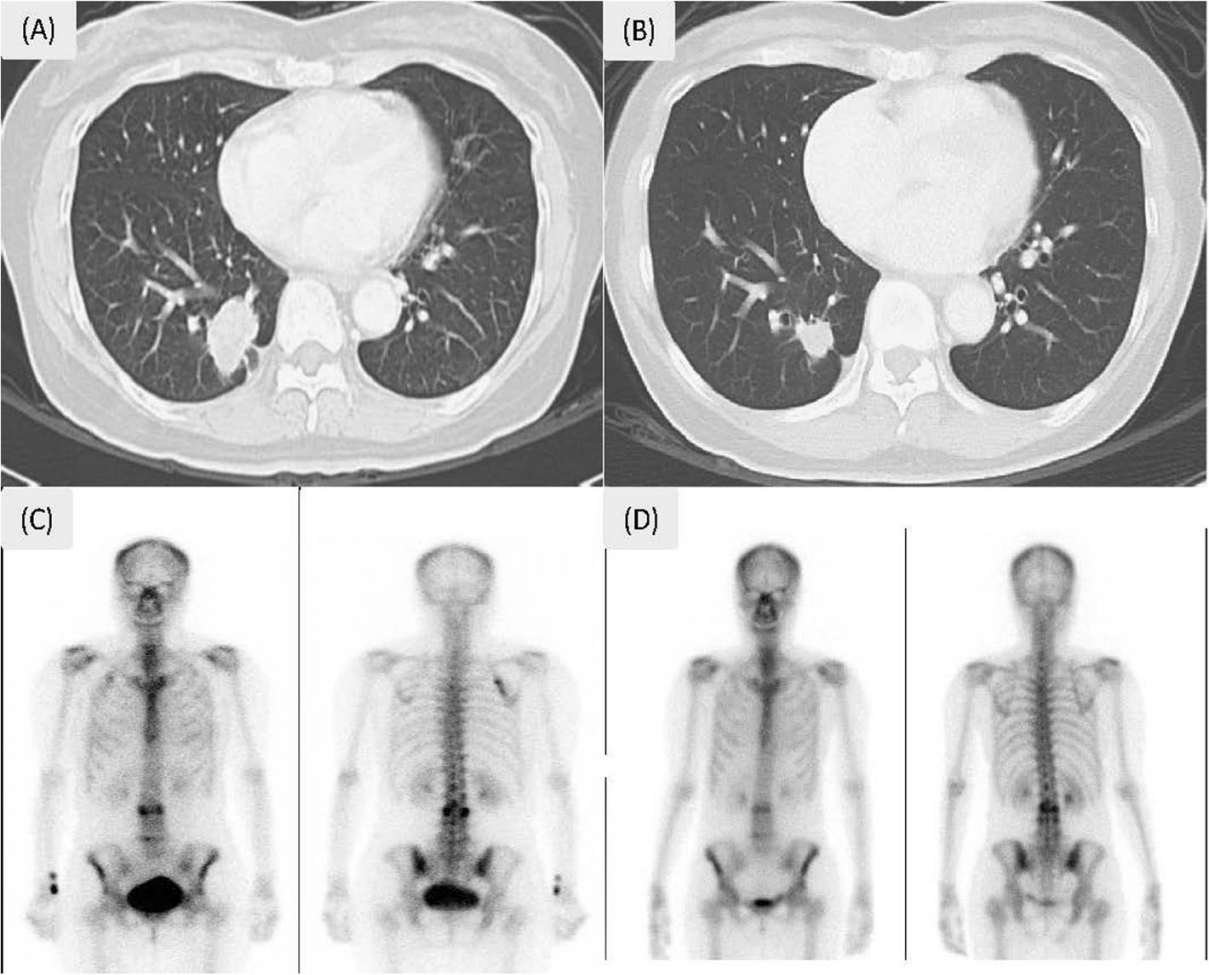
Patient scans. a Chest computed tomography scan: pre-tyrosine kinase inhibitors (TKI) therapy in March 2017. b Post-TKI therapy and pre-surgery in November 2018; right lower lobe lung mass regressed but noticeable. c Whole body bone scan: pre-TKI in March 2017. d During TKI therapy in July 2018
